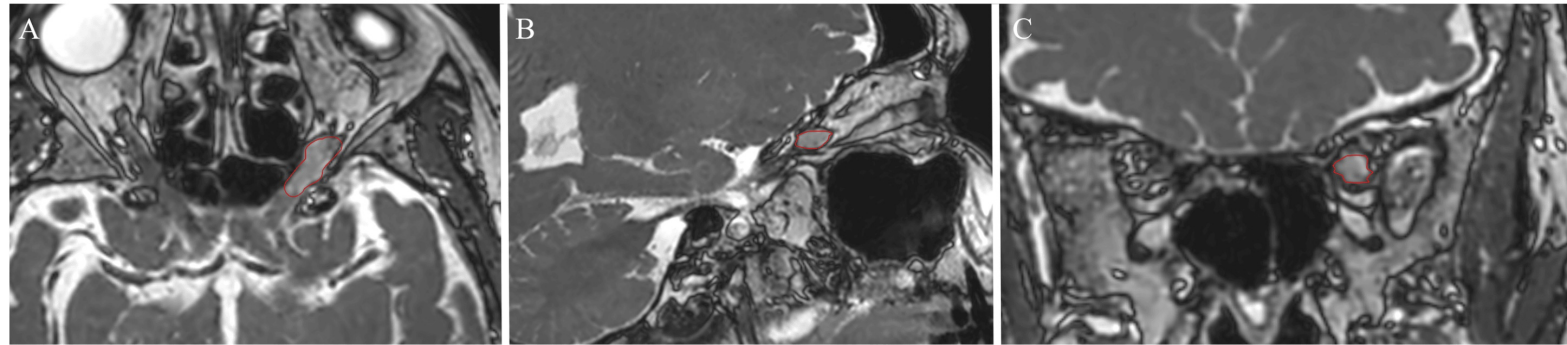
Pre-treatment SSFP MRI: A) axial section, B) sagittal section, and C) coronal section (ONSM shown in red). The tumor displayed an increased fluid signal in the left optic nerve sheath with edema. Image Credit: Michael Chaga SSFP: steady-state free precession; ONSM: optic nerve sheath meningioma
